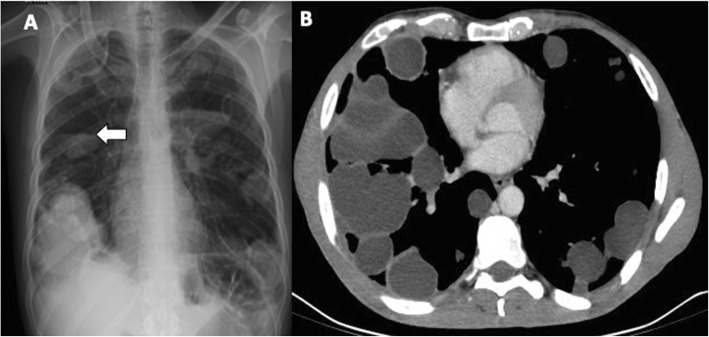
A 50-year-old man with hepatic and pleuro-pulmonary echinococcosis, secondary to trans-diaphragmatic dissemination. a Chest x-ray shows bilaterally multiple round cavitated lesions—some of which with air-fluid level (arrow)—as well as radiopaque lesions. b Chest CT scan of the same patient shows multiple cystic lesions, some of which appear stuffed with fluid-density material, others cavitated with air-fluid levels (not shown in this image)
